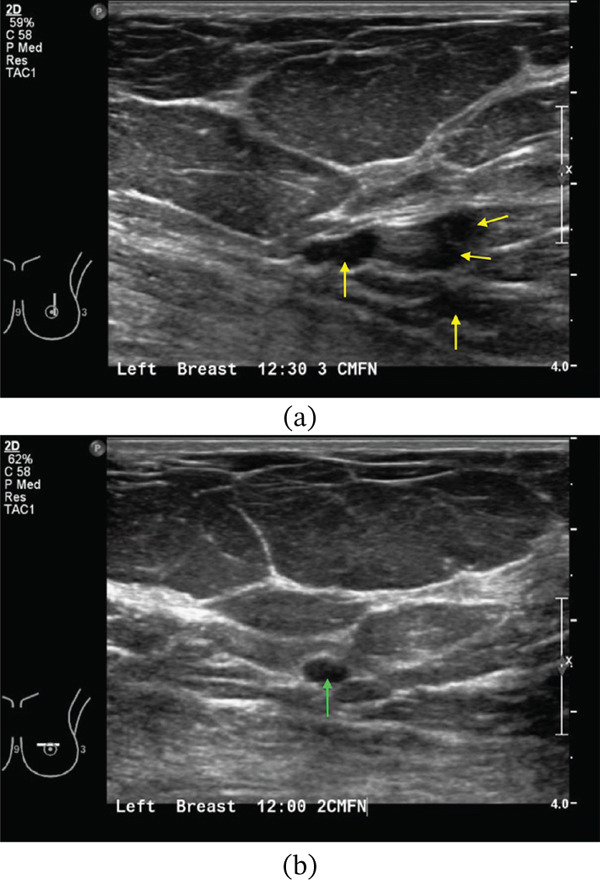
Ultrasound of the subareolar left breast in (a) radial and (b) antiradial projections demonstrates multiple complex cystic/solid masses at the 12 o′clock position ranging from 4 to 8 mm (yellow and green arrows). BI‐RADS 3 (probably benign).
